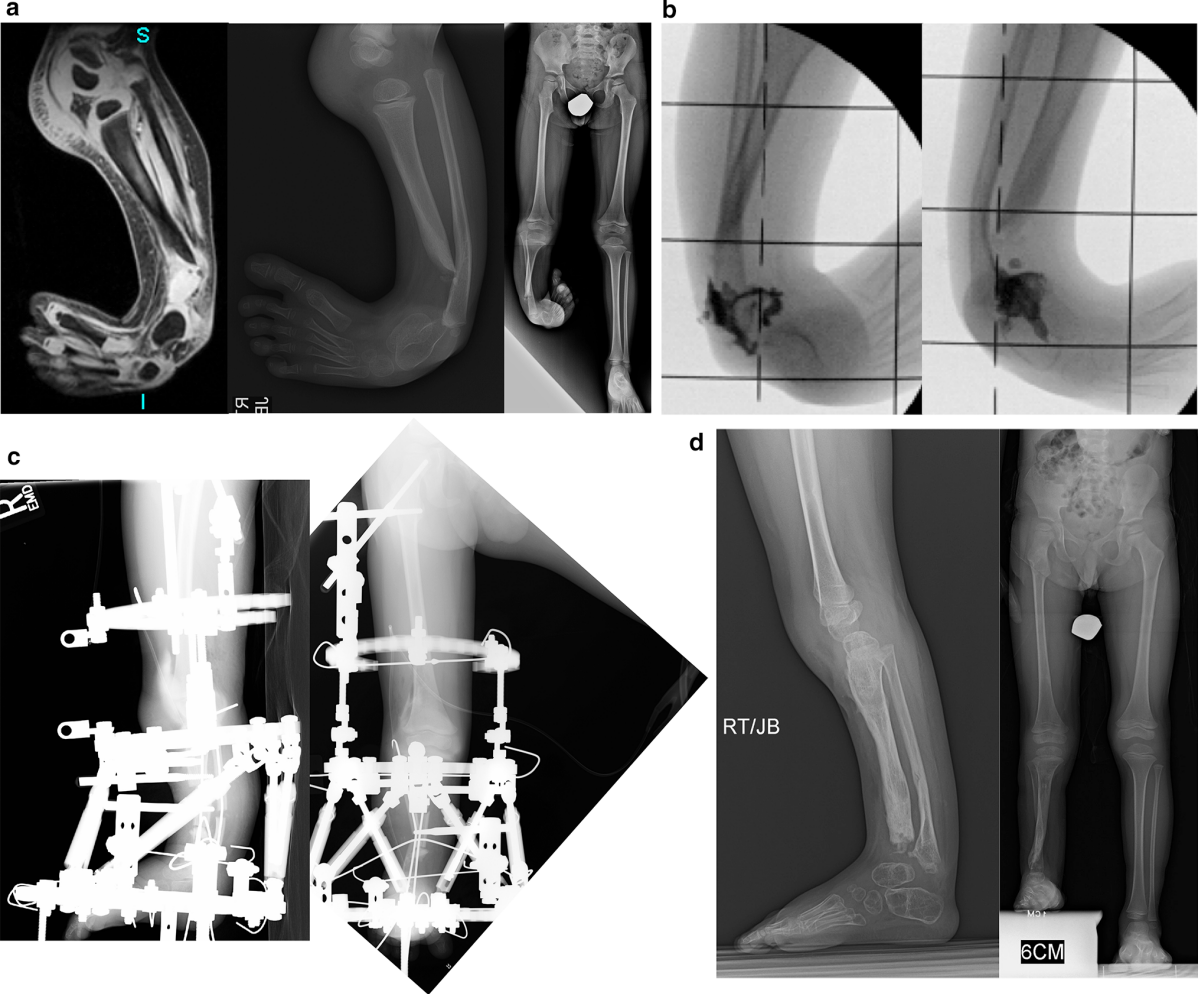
a Magnetic resonance imaging (MRI) scan (*left*), lateral radiograph (*middle*), and standing AP radiograph (*right*) showing Paley type 2C unilateral tibial hemimelia. The foot is in significant varus. The distal tibia has an unossified region contiguous with the ankle. There is no obvious distal tibial physis, but the plafond is present. Note that the ossification of the distal tibia is irregular and sloped, not like a normal metaphysis associated with a distal tibial physis (Fig. 5a). The proximal fibula is relatively overgrown and proximally migrated. b Arthrogram of ankle showing AP (*left*) and lateral (*right*) views. c Lateral (*left*) and AP (*right*) radiographs after arthrotomy of ankle, and open valgus-extension osteotomy and shortening of distal tibia to realign ankle joint by acute correction. Bone morphogenetic protein 2 (BMP2) was inserted in drill holes of the cartilage remnant of the distal tibia to get it to ossify. A second osteotomy was performed proximally for lengthening. The fibula is being pulled distally with an intramedullary wire hooked over the proximal epiphysis (see AP view). Because the tibial/ankle/foot deformities were corrected acutely, the external fixator is programmed for pure lengthening. The external fixator is extended to the femur with knee hinges to protect the cruciate deficient knee during lengthening while permitting knee flexion and extension motion. d Lateral (*left*) and AP (*right*) radiographs, after lengthening with excellent consolidation of the tibia, including ossification of the delayed distal tibia. The foot is plantigrade with forefoot supination deformity present. The proximal fibula is at station
